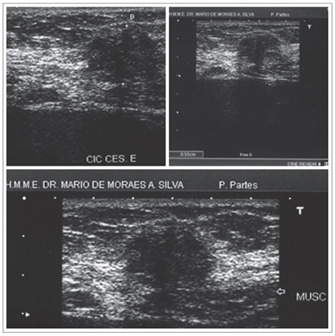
Ultrasound scan on a case of endometrioma in the abdominal wall (cesarean section scar). Details show hypoechoic image with posterior acoustic shadow.
